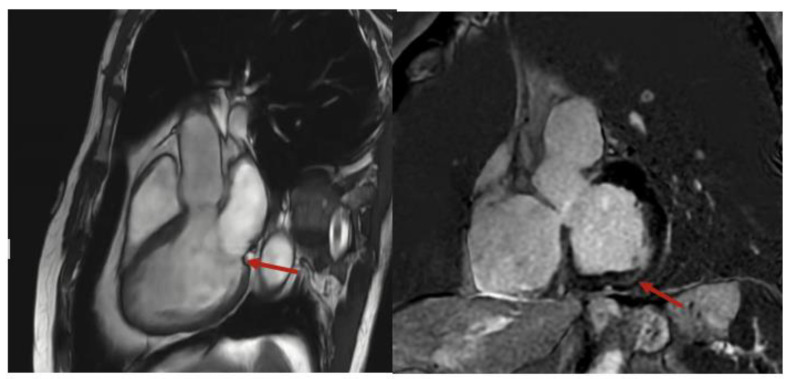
Cardiac magnetic resonance imaging with mitral annular disjunction in sagittal (left) and short axis (right) views. Red arrow indicates area of mitral annular disjunction and scarring, respectively.
